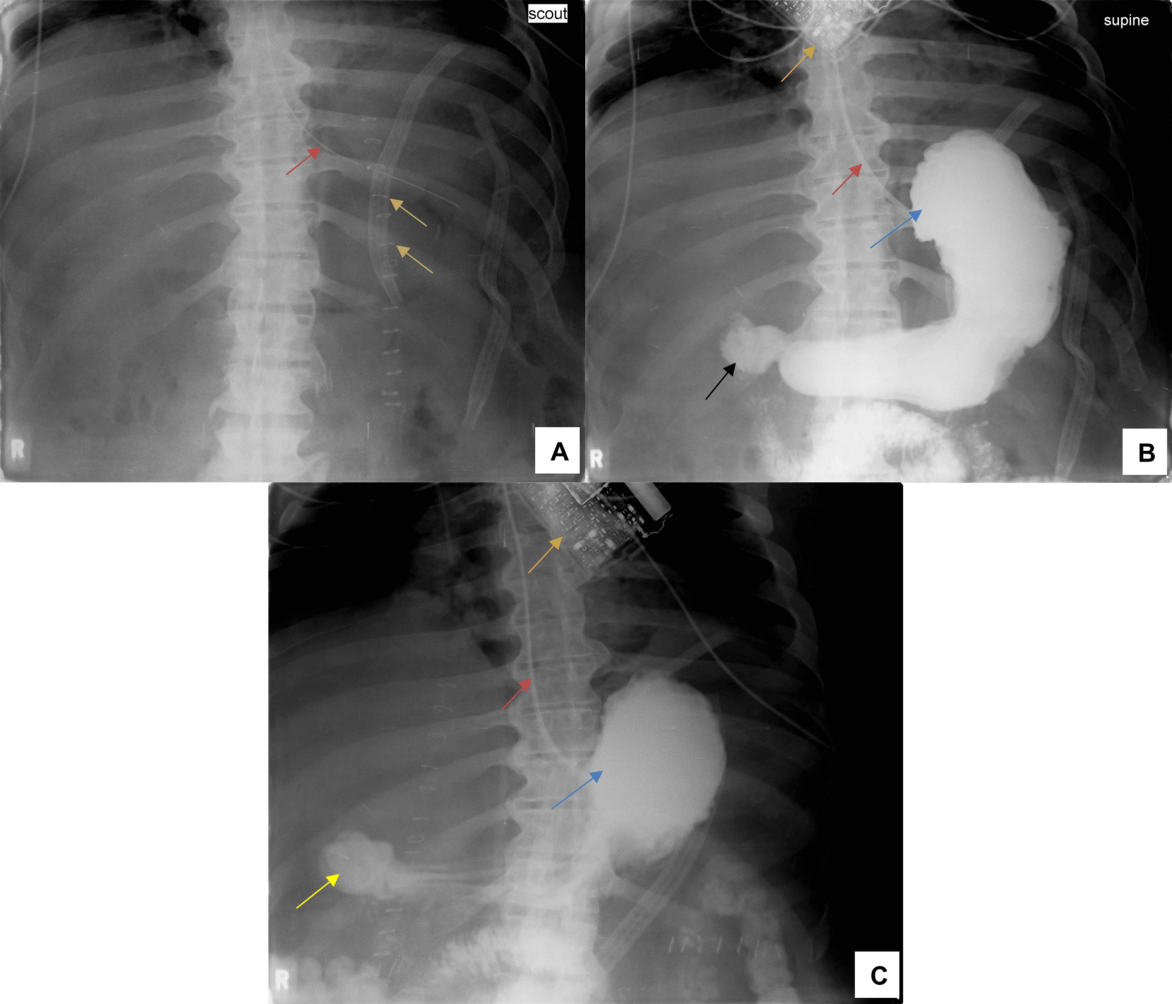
Supine UGI radiograph demonstrating postoperative anatomy following laparoscopic gastric band removal A: Scout abdominal radiograph prior to contrast administration. A radiopaque enteric NG tube is visualized coursing along the esophagus toward the stomach (red arrow). Metal clips can be seen near the gastroesophageal junction (yellow arrows). No pneumoperitoneum or abnormal bowel gas patterns are identified. B: Upper GI contrast study AP view following administration of Gastrografin. The contrast-filled gastric lumen is clearly visualized (blue arrow), with smooth filling of the gastric body and antrum. Contrast passes normally into the duodenal bulb (black arrow). The orange arrow shows the lap band device (no longer in its normal position). No extraluminal contrast is seen, and the absence of leak is noted. Position of the NG tube (red arrow). C: The stomach remains uniformly opacified (blue arrow), with unobstructed flow into the duodenal sweep. The non-opacified excluded gastric remnant persists in its expected location (yellow arrow), consistent with intact anatomy and no fistulous communication. No extraluminal contrast is identified. The red arrow indicates the position of the NG tube. The orange arrow indicates the lap band device, no longer connected. UGI, upper gastrointestinal; NG, nasogastric; GI, gastrointestinal; AP, anteroposterior
